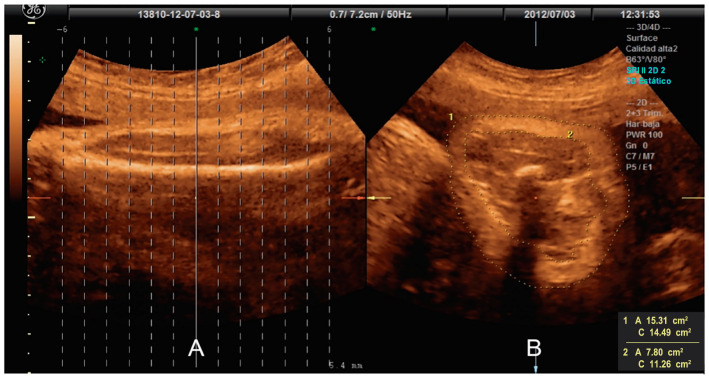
Offline Tomographic Ultrasound Imaging analysis. (A) Sagittal plane of the fetal femur. (B) Axial plane selected, fat area obtained by subtracting the lean tissue area (muscle and bone) from the total area (covering the total area).
